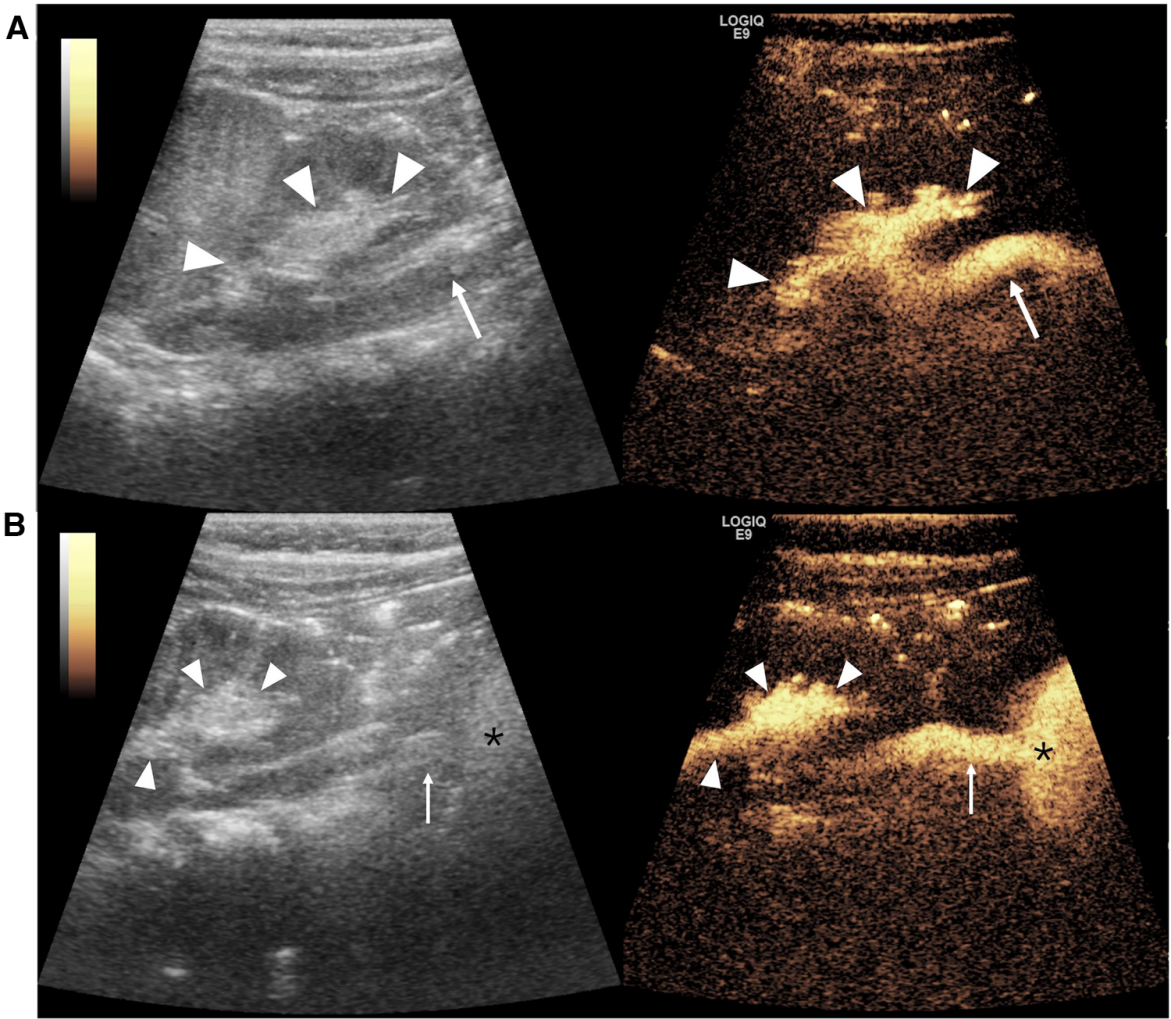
Vesicoureteral reflux imaging on contrast-enhanced voiding urosonography of the dual-screen mode of the left kidney in a 1-year-old boy. (A) The sagittal plane of the left kidney displays contrast agent refluxing into the ureter (arrow) and pelvicalyceal system (arrowheads), indicating grade III reflux. (B) Panoramic coronal view image: simultaneous visualization of the left kidney (arrowheads), ureter (arrow), and bladder (asterisk) from the left flank full of echogenic microbubbles in a single view.
